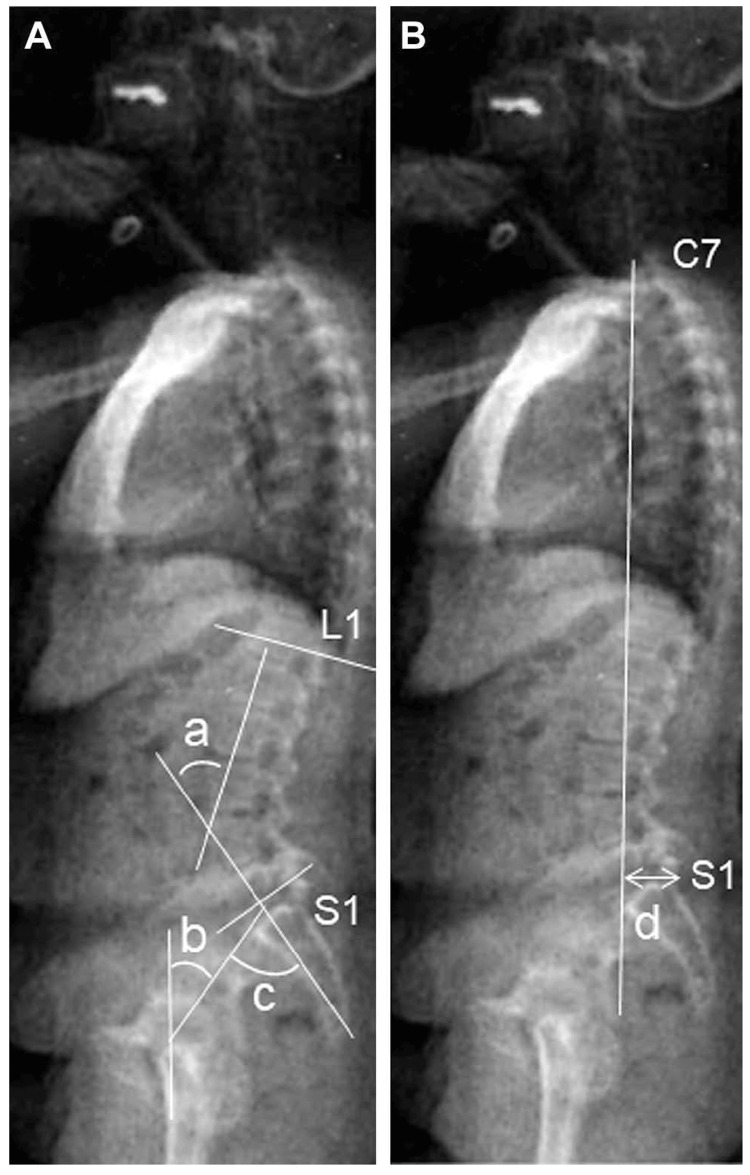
Radiographic assessment of sagittal spinal and pelvic parameters. Parameters measured on standing lateral radiographs of the whole spine and pelvis include: In panel A, (a) Lumbar lordosis: Cobb angle from the upper endplate of L1 to the lower endplate of S1; (b) Pelvic tilt: angle between the line connecting the midpoint of the sacral plate to the axis of the femoral heads and the vertical axis; (c) Pelvic incidence: angle between the line perpendicular to the sacral plate at its midpoint and the line connecting this point to the axis of the femoral heads; in panel B, (d) Sagittal vertical axis (SVA): horizontal distance from the C7 plumb line (originating at the middle of the C7 vertebral body) to the posterior superior endplate of S1. This figure was created using images and descriptions for which we retain the copyright, based on ref [15].
